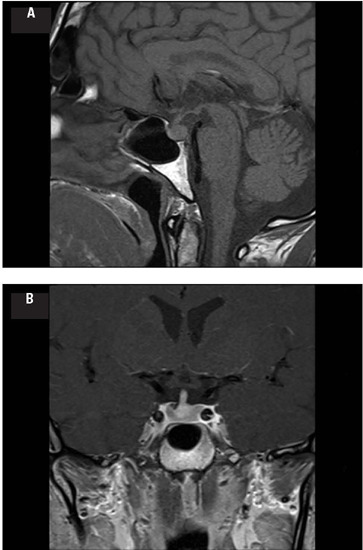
Pituitary magnetic resonance imaging (MRI) T1-weighted images showing
